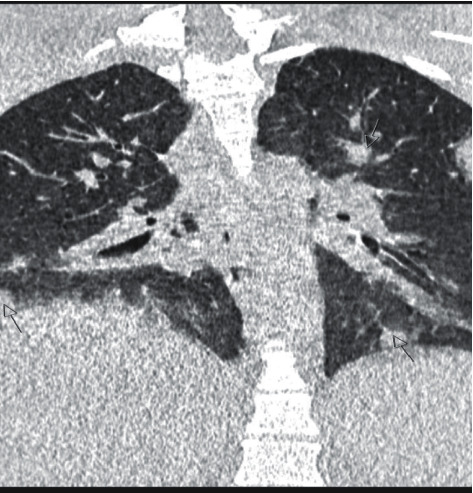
Chest computed tomography scan, coronal reconstruction, shows multiple bilateral nodular pulmonary consolidations (arrows).
